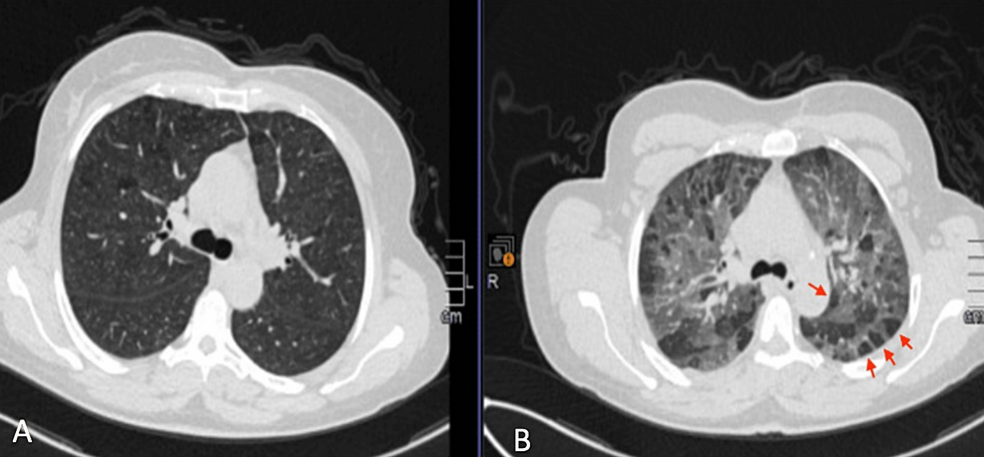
Chest CT scan in the parenchymal window showing a mosaic aspect. High-resolution chest CT scan sections in inspiration (A) and forced expiration (B) with MINI IP reconstruction showing a mosaic aspect with expiratory trapping (>5 lobules per lobe) and a "head cheese" aspect.
